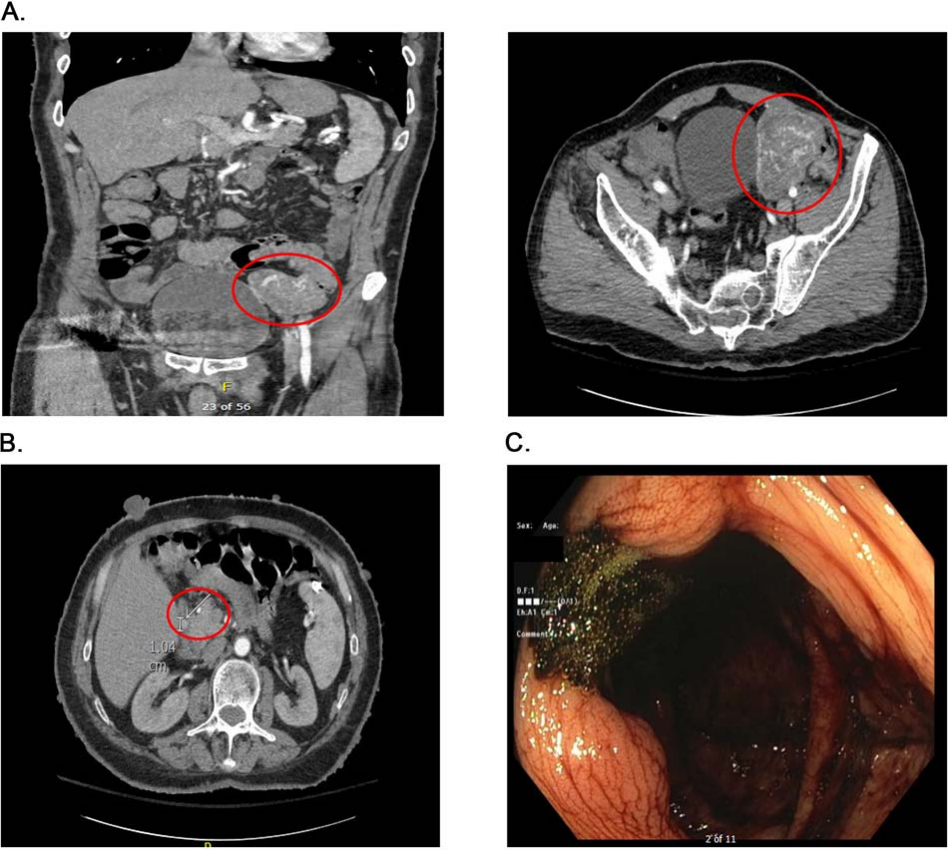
Presurgical diagnostics. (A) CT imaging of the large jejunal mass. (B) CT imaging of small duodenal mass. (C) Blood at the ileo-cecal valve.
